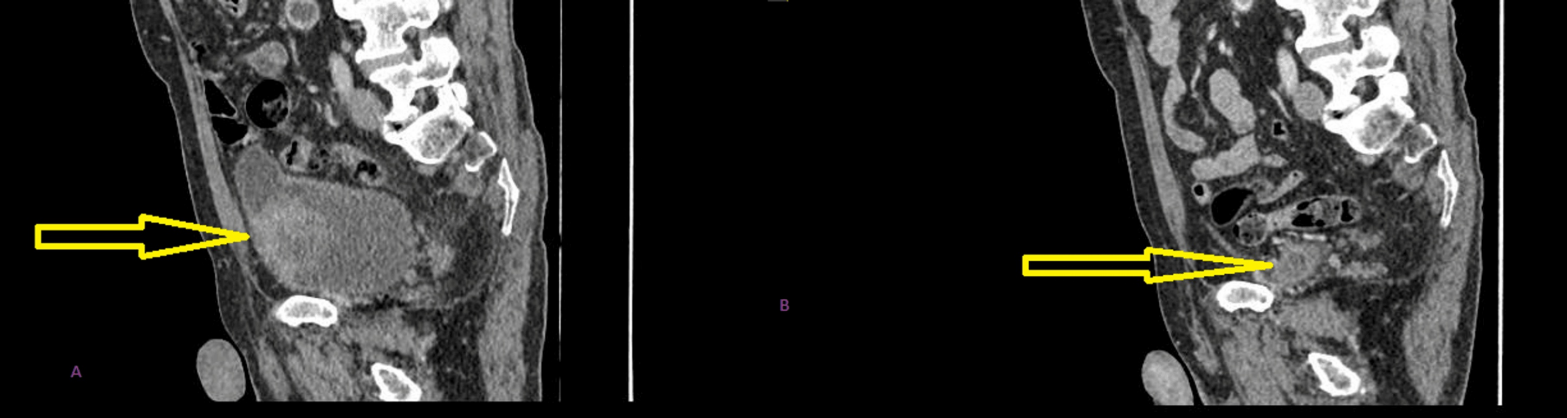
Sagittal view of CT scan before TURBT in figure A and after TURBT but prior to radiotherapy with urethral catheter in situ in figure B. The bladder mass is seen clearly in figure A, whereas in figure B, the mass can no longer be seen following TURBT. TURBT - transurethral resection of bladder tumor
